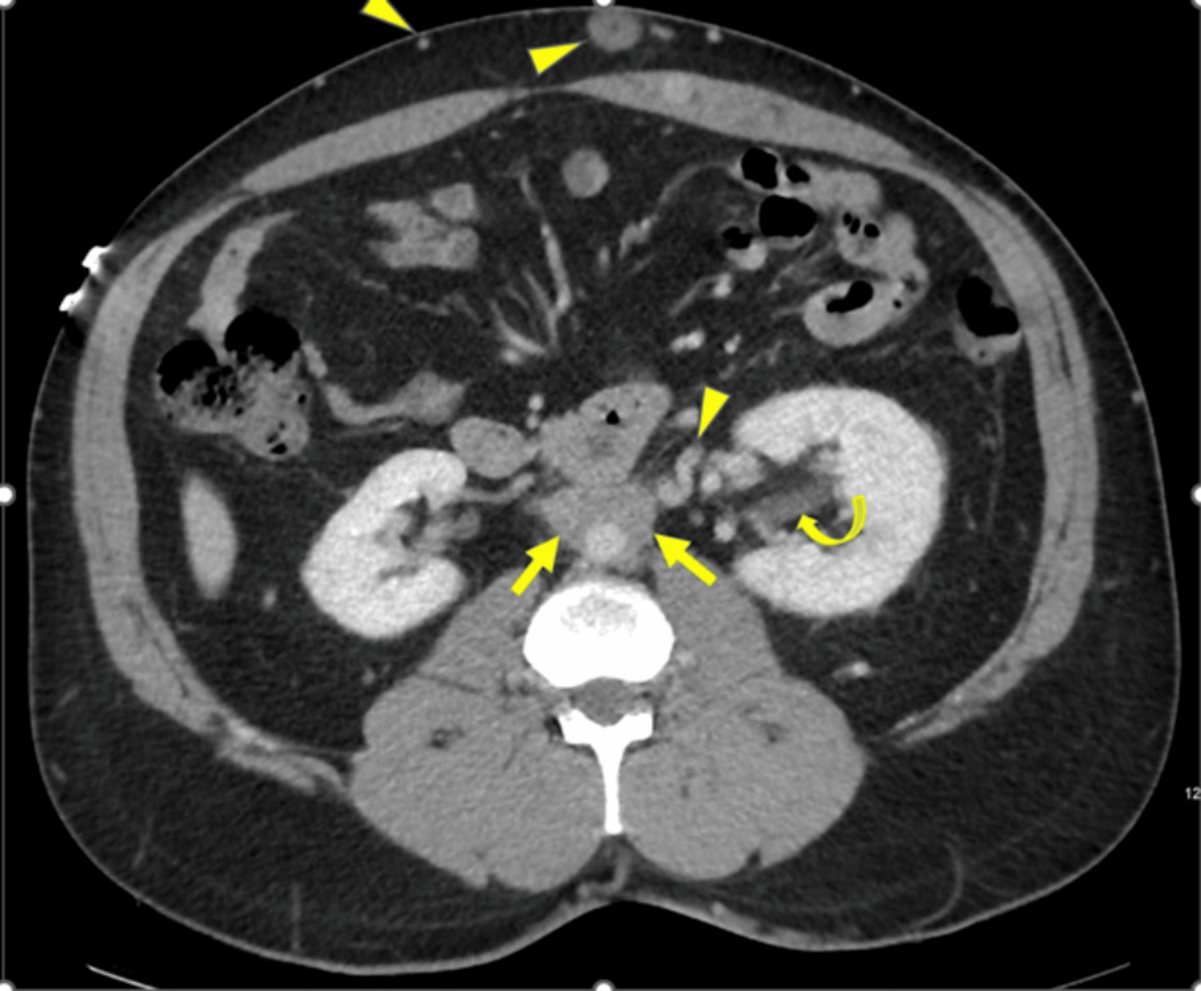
Axial CT image shows periaortic soft tissue (bold arrows), severe compression of IVC (not seen) with multiple retroperitoneal and subcutaneous venous collaterals (arrowheads), compressing the ureters and left hydronephrosis (curved arrow) CT-Computed Tomography IVC- Inferior Vena Cava
